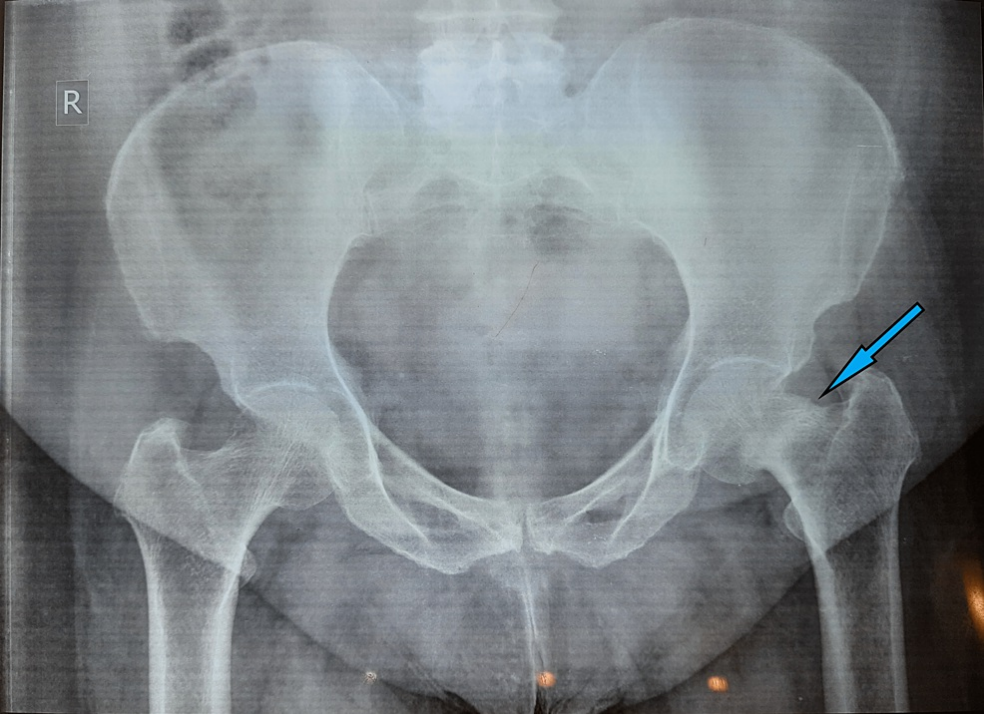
A six-month follow-up pelvic X-ray, showing healed fracture of the left femur neck stress fracture after nonoperative treatment
